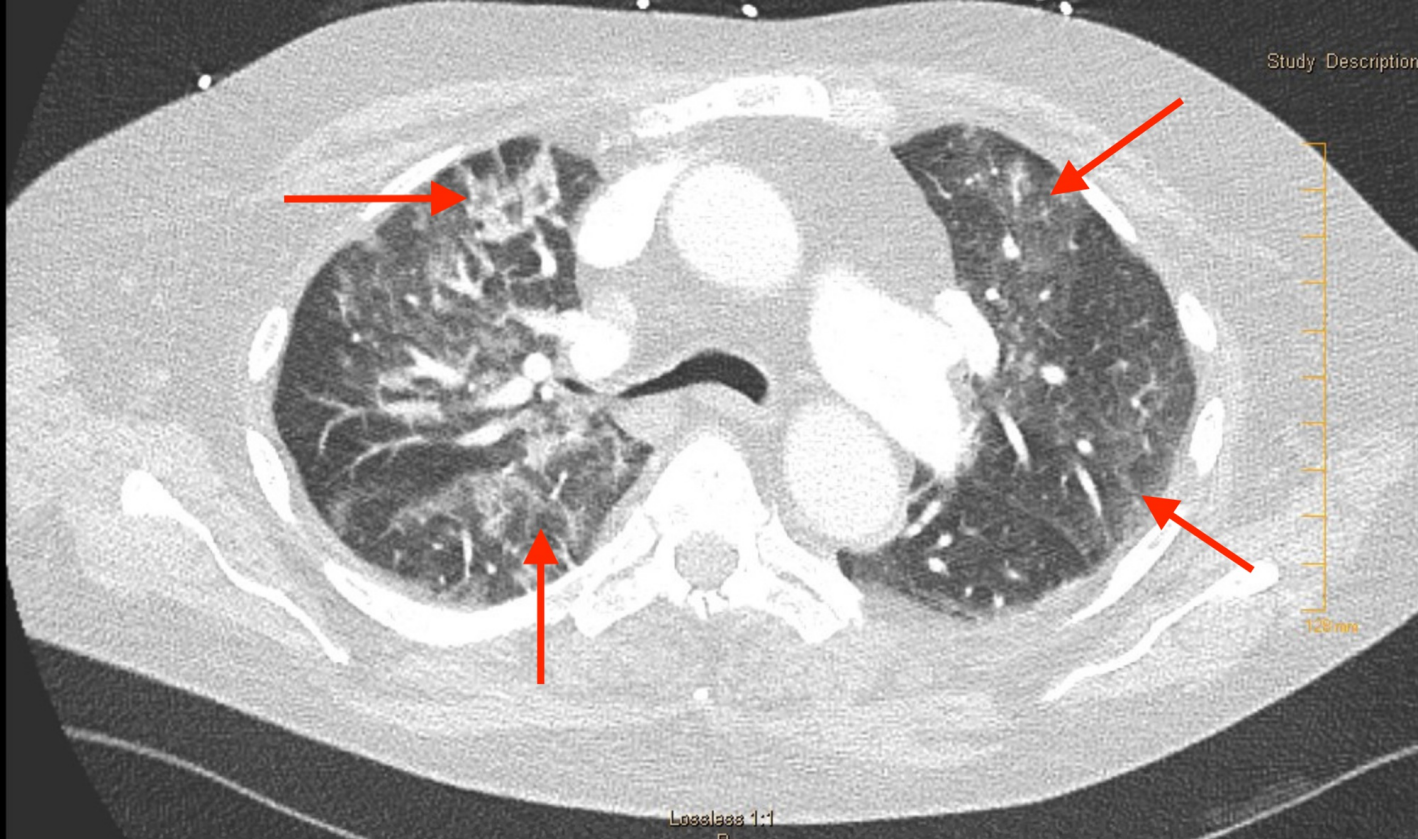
Axial contrast-enhanced CT chest (lung window) showing bilateral diffuse ground-glass opacities.
